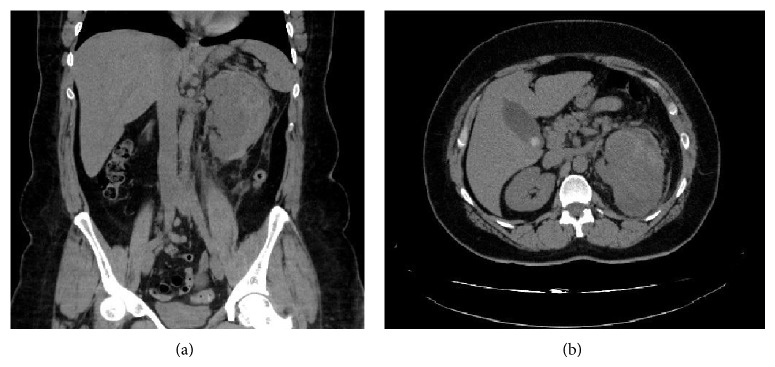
(a) The coronal reconstructed CT image shows a large solid heterogeneous mass without calcifications in the left kidney and also a perirenal hematoma. (b) The axial CT image of the upper abdomen reveals a large solid heterogeneous mass measuring 7 × 6.7 in the left kidney and also a perirenal hematoma.
